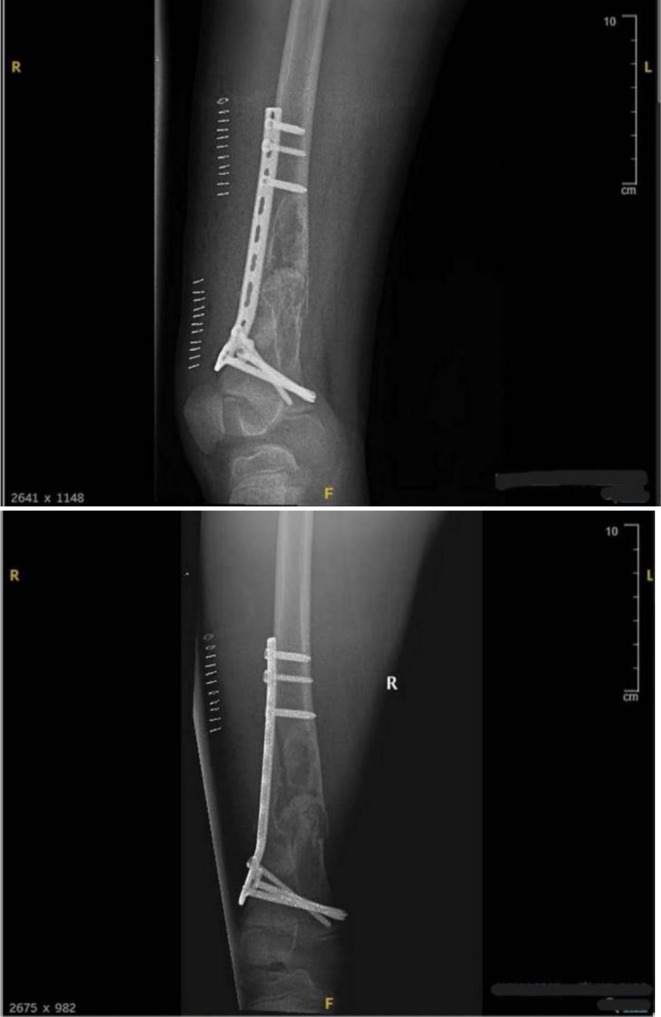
Early postoperative anteroposterior and lateral radiographs showing distal femoral fixation and grafted curettage defect. The distal femoral pathological fracture demonstrates maintained alignment after ORIF with plate‐and‐screw fixation and bone grafting of the curetted lesion cavity. No implant malposition is seen. Technical note: The lateral view is suboptimal due to pain‐limited positioning.
